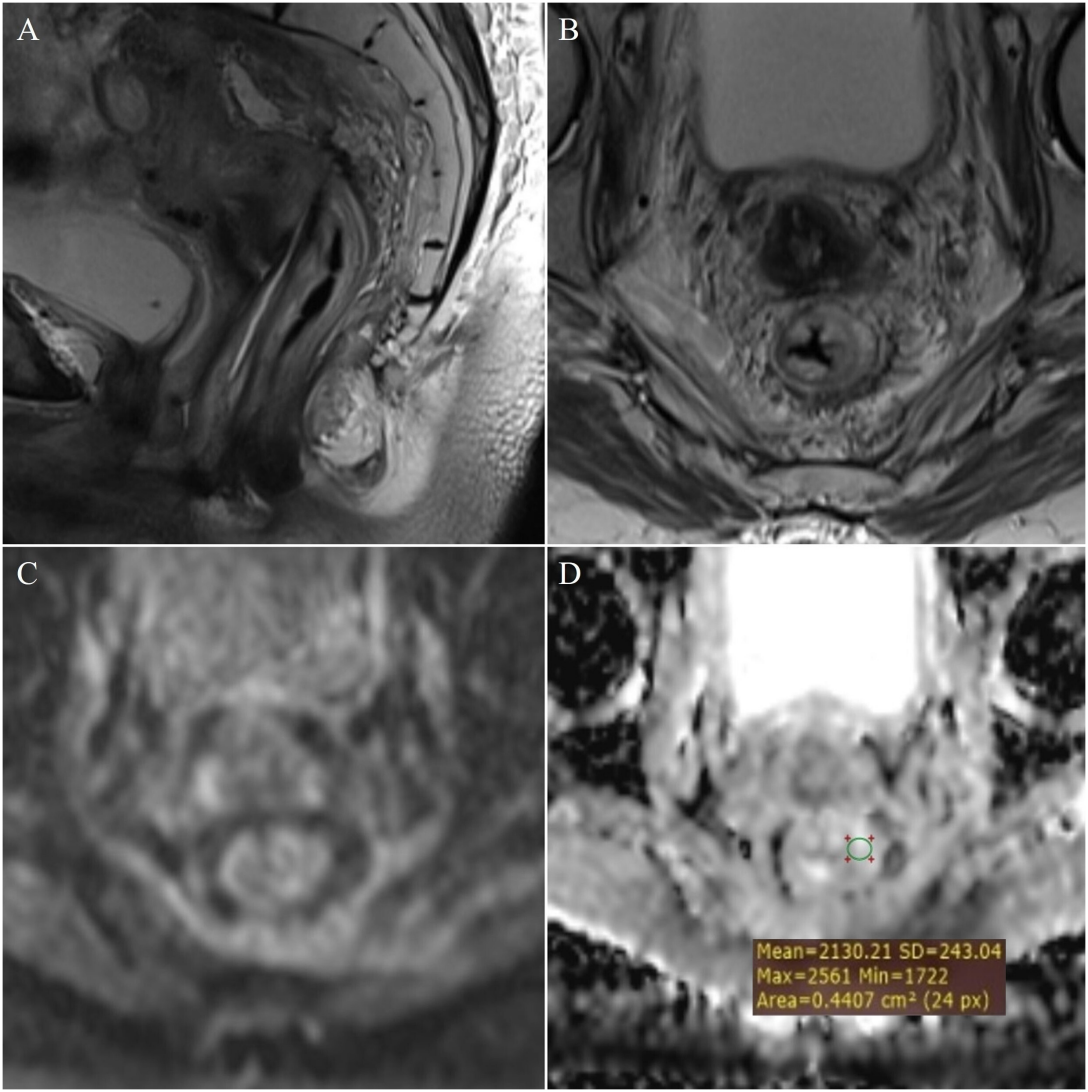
Measurement of ADC values: post-radiation proctitis - (A) (T2W sagittal plane), (B) (T2W axial plane), (C) (DWI) and (D) (ADC map with ROI).
